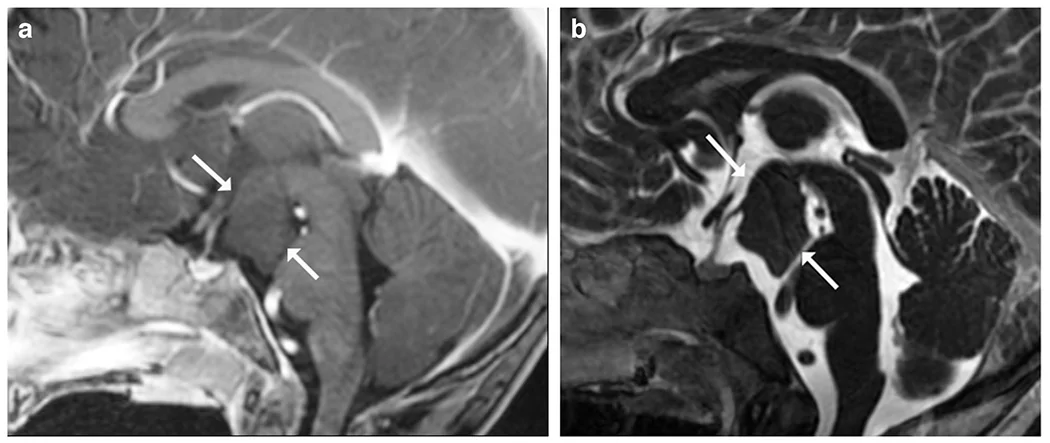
Three-year-old female with gelastic seizures and intrahypothalamic (sessile) hamartoma. a Sagittal T1-weighted post-contrast and (b) sagittal volumetric T2-weighted steady-state acquisition images show a non-enhancing and uniformly isointense hamartoma (*arrows*) centered at the level of the hypothalamus
